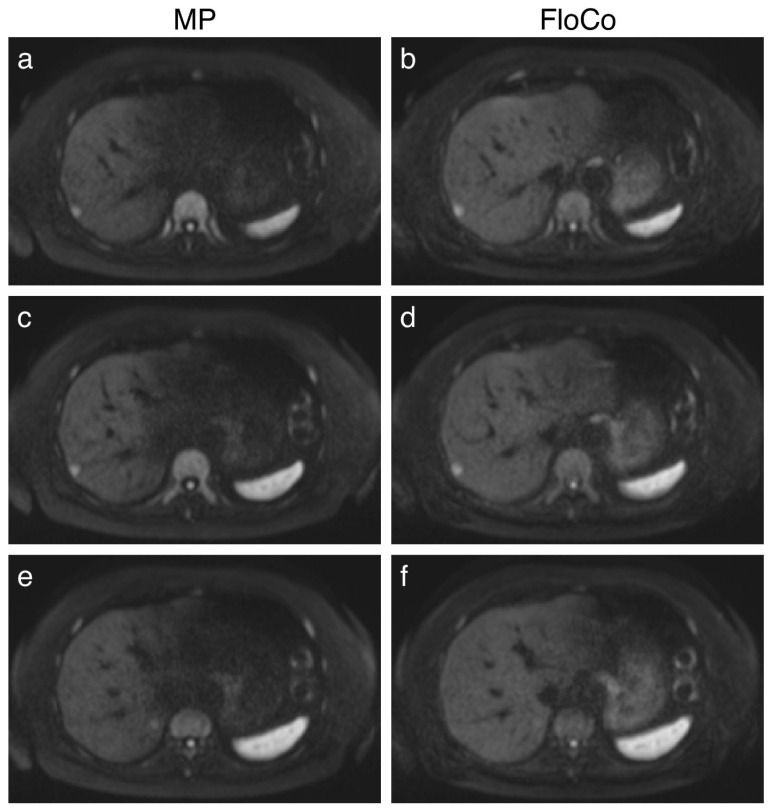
Liver diffusion-weighted MRI (b = 800 s/mm^2^) of a patient with metastatic colorectal carcinoma. Examples of signal dropout. (a,c,e) Monopolar (MP) DWI, three adjacent slices; (b,d,f) flow-compensated (FloCo) DWI, three adjacent slices. Note the reduced ability to determine the liver’s border due to signal dropout in the MP images. Note that a lesion is more clearly visible in the MP image (e) than in the corresponding FloCo image (f), consistent with our finding that in some cases MP provided greater lesion conspicuity.
